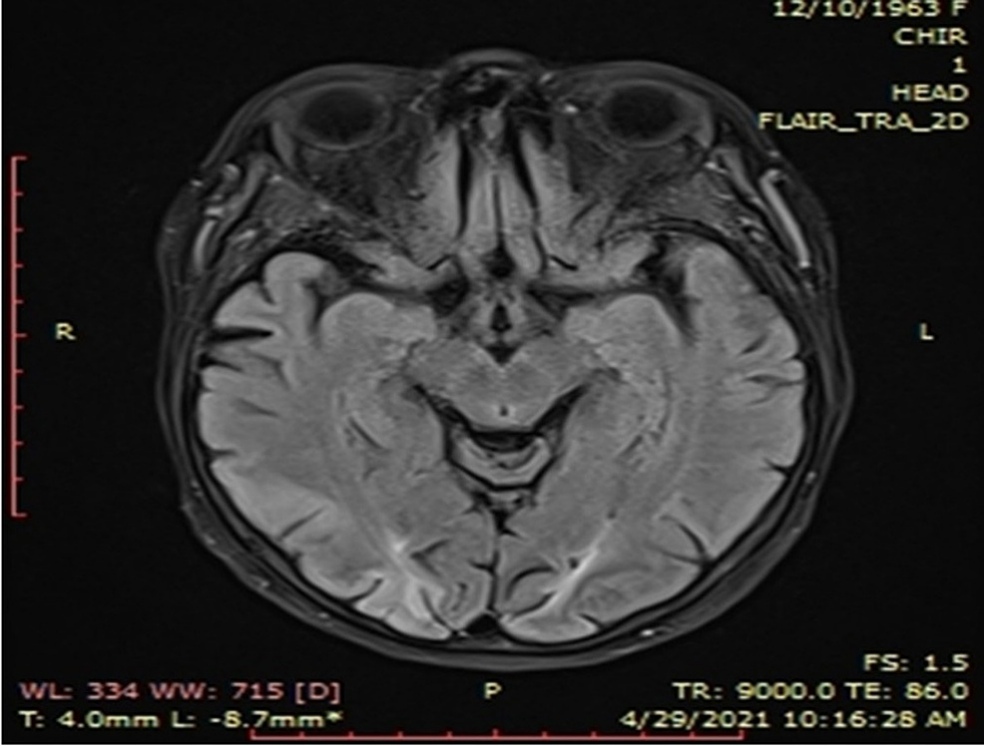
Head MRI scan showing bilateral parieto-occipito-frontal subacute cortical ischemia
